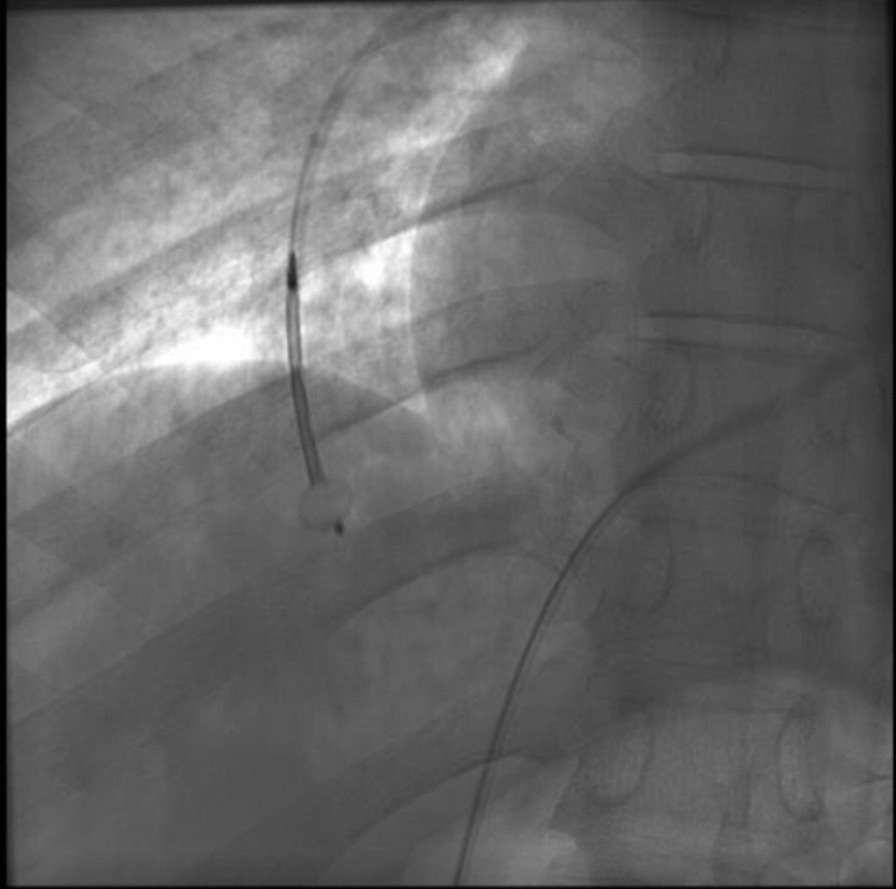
Computed tomography angiogram image pre-embolization of the vasculature feeding pulmonary arteriovenous malformation
